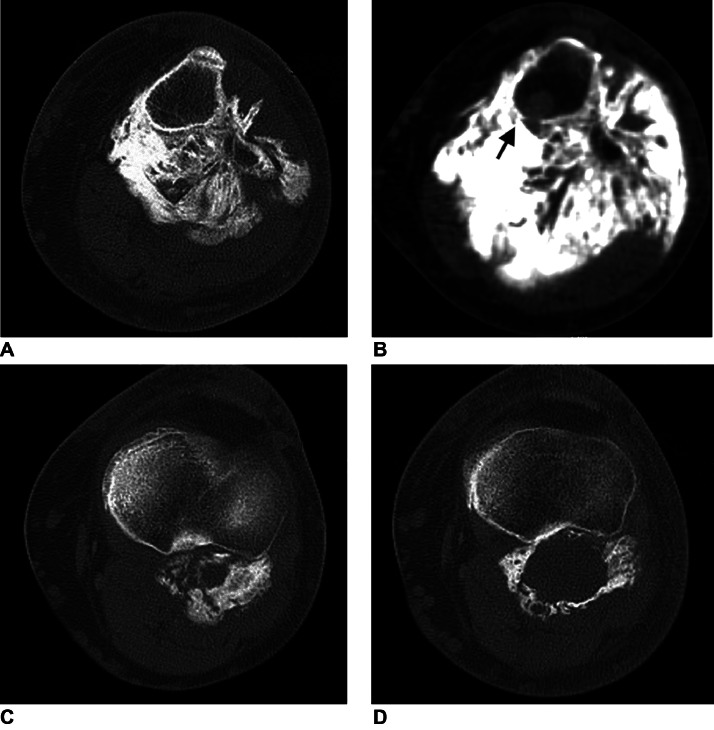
Comparison of initial (A, C) and subsequent (B, D) CT axial scans. Images show progression of size of the mass and adjacent soft-tissue areas and signs of invasion to bone marrow of tibial metaphysis (arrow).
